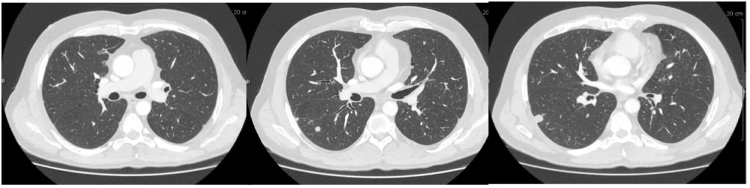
Patient’s chest CT scan 2 months after the unique intramuscular injection of benzathine penicillin G (2.4 million units).
